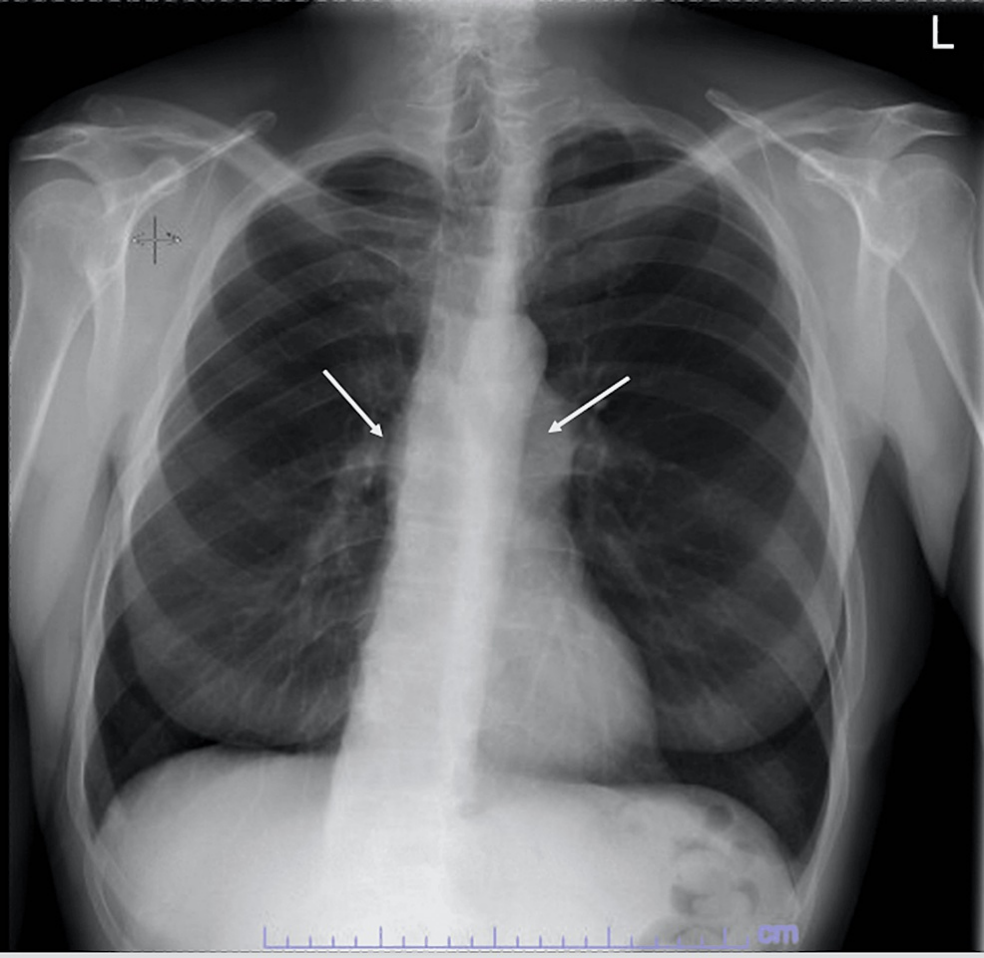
X-ray suggestive of mild enlargement of the proximal pulmonary arteries.
